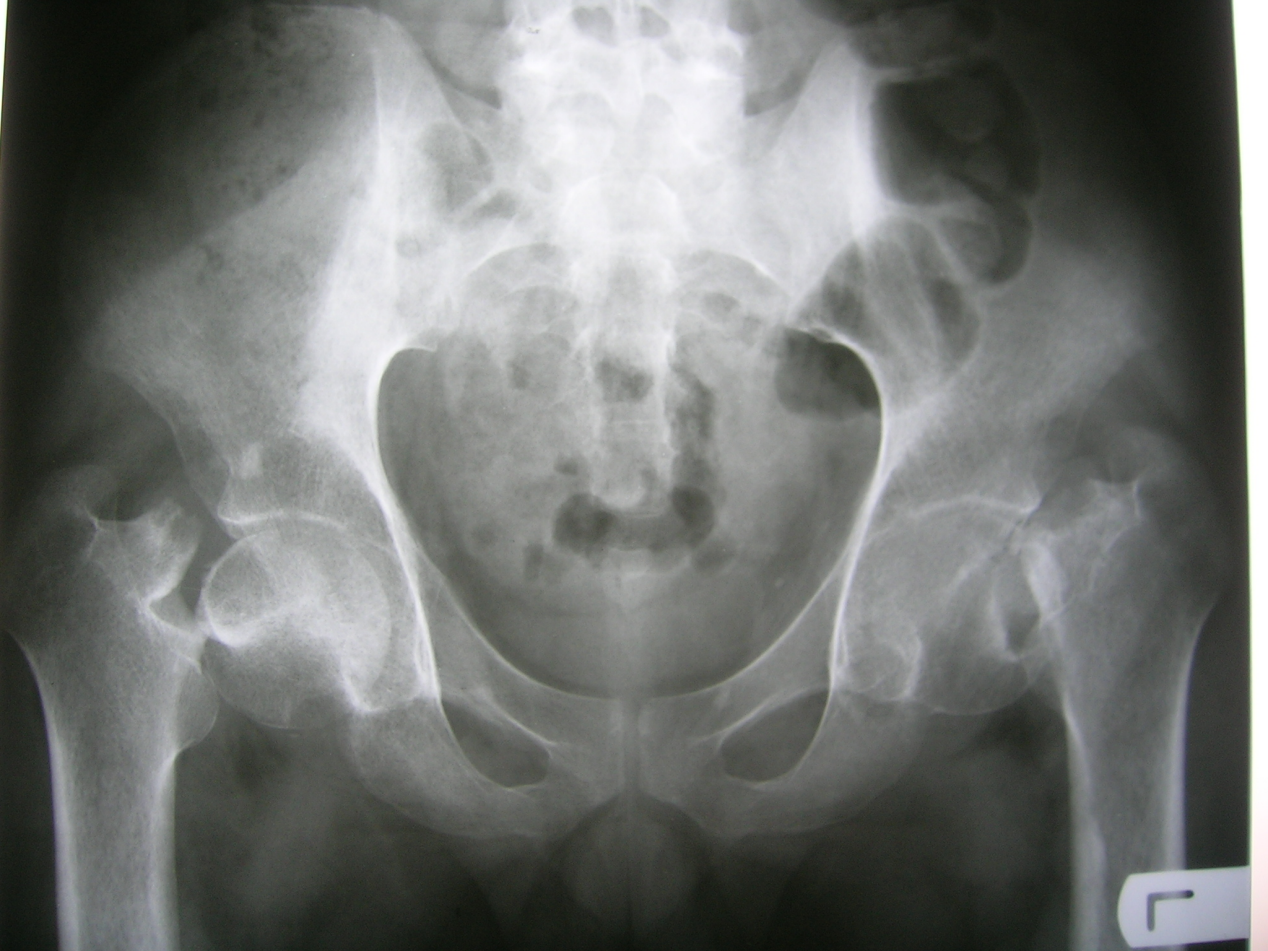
Radiograph taken before operation demonstrates displaced bilateral femoral neck stress fractures.
